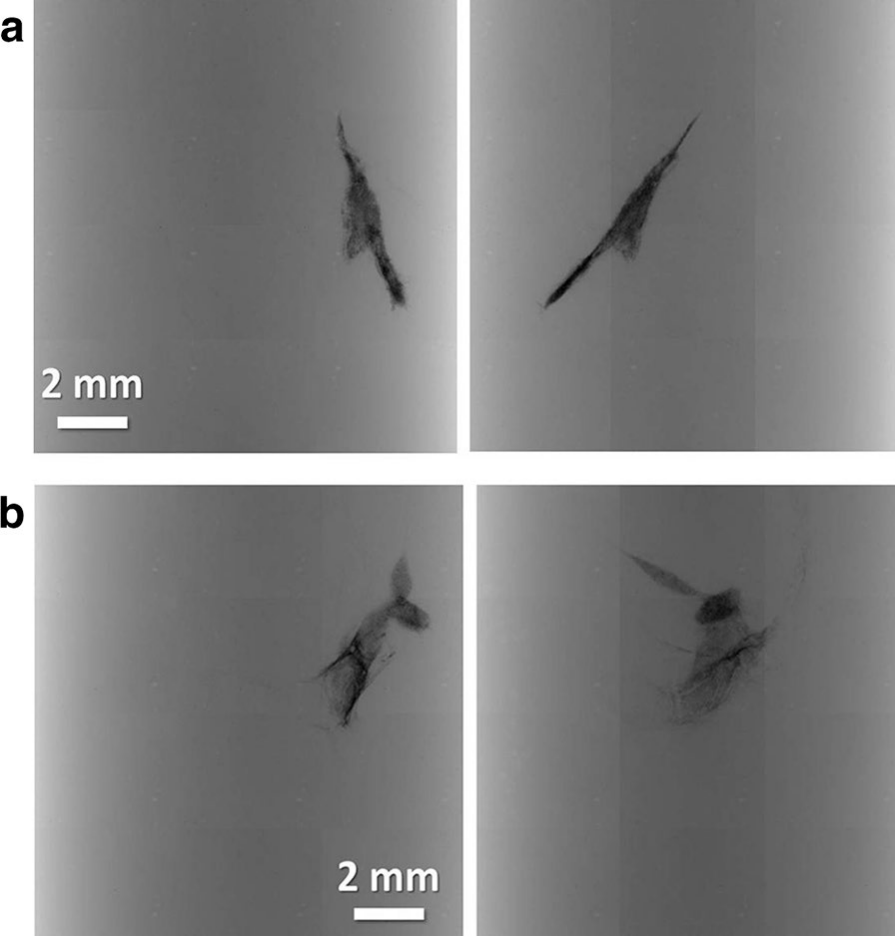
Projection x-ray images of tumor cells loaded with bare AuNPs in the brain, from two examples (a and b) each of two different points of views: anteroposterior and lateral. The black dots correspond to tumor cells
